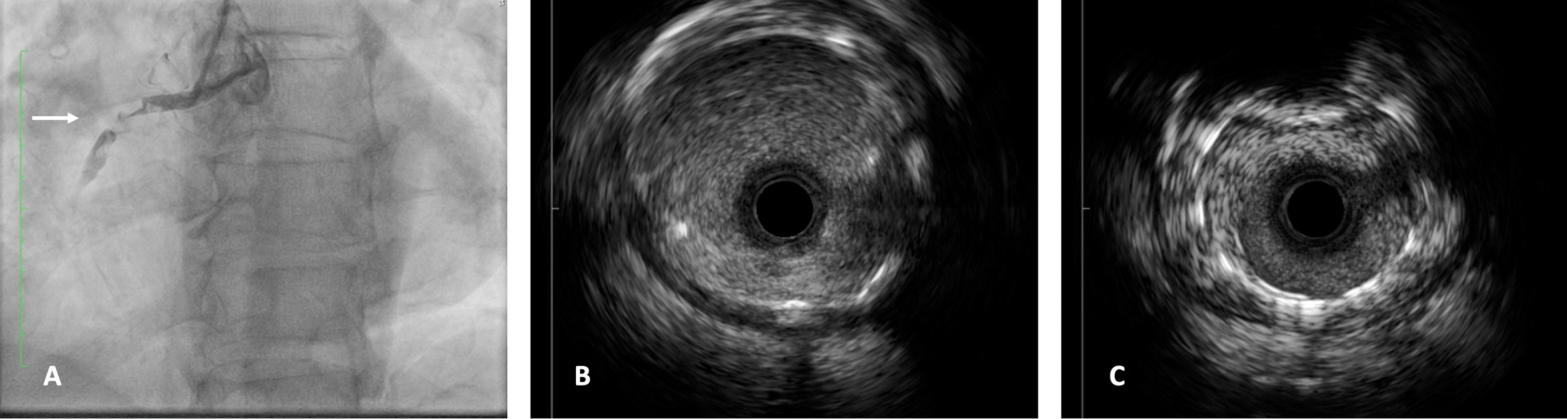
Diagnostic coronary arteriography and IVUS findings of the RCA (A) Diagnostic coronary arteriography: fluoroscopy showing a lesion in the proximal third of the right coronary artery (white arrow). (B) IVUS demonstrating stent restenosis in the distal third of the RCA. (C) IVUS image revealing thrombus formation in the proximal segment of the RCA IVUS: intravascular ultrasound; RCA: right coronary artery
